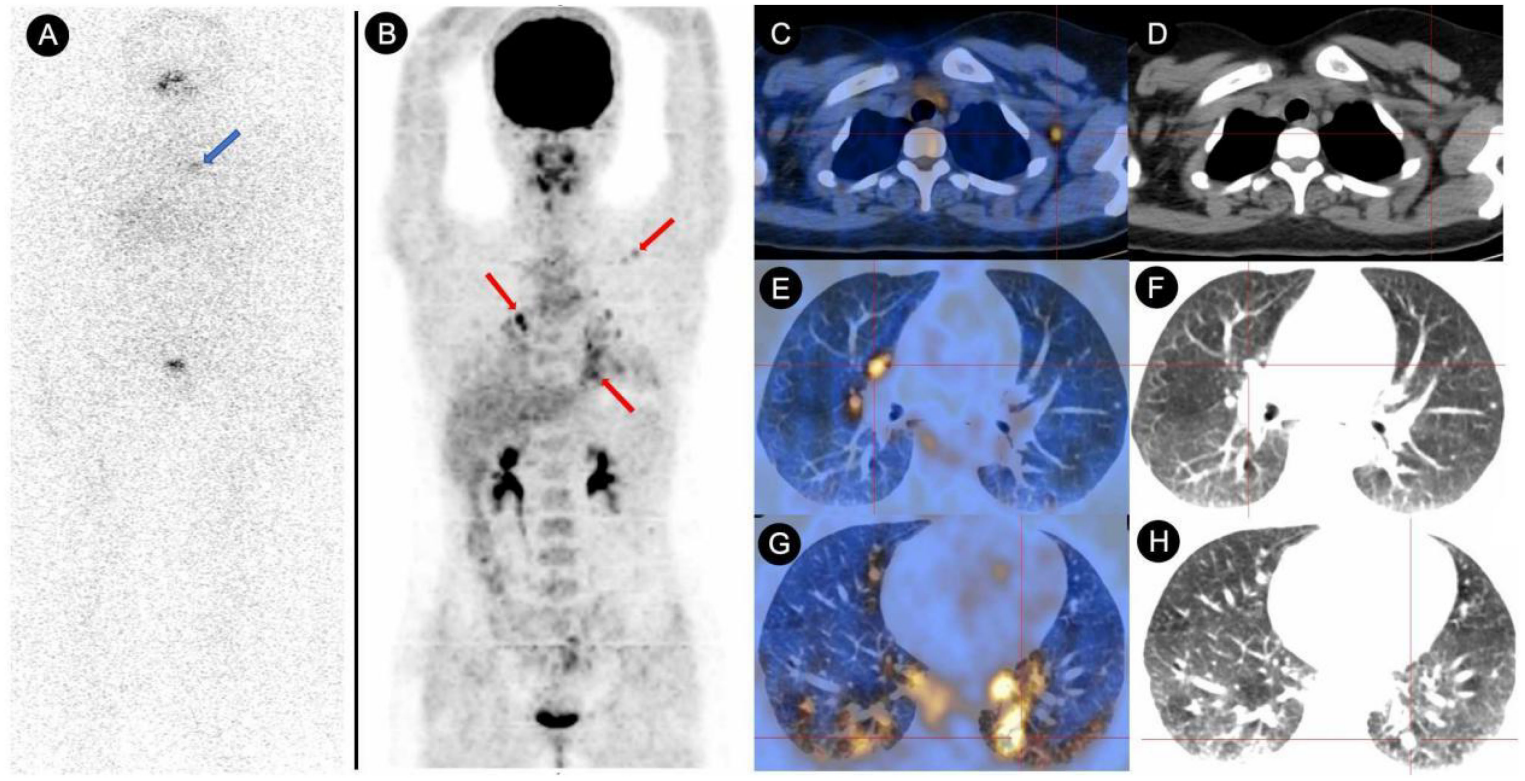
Comparative analysis of ^131^I-WBS and ^18^F-FDG PET/CT in a DTC patient with persistently elevated TgAb (>4000 IU/mL) over a 48-month follow-up after ^131^I treatment. (A) The 131I-WBS revealed no uptake of ^131^I in any lesions throughout the body (the area indicated by the blue arrow showed abnormal accumulation of ^131^I, which was confirmed by SPECT/CT to be physiological uptake by the thymus). (B–H) The whole-body MIP (Maximum Intensity Projection) image from the ^18^F-FDG PET/CT scan, as well as the fused PET/CT and CT images of metastatic lesions, indicating multiple lymph node metastases and lung metastases (red arrow and cross).
